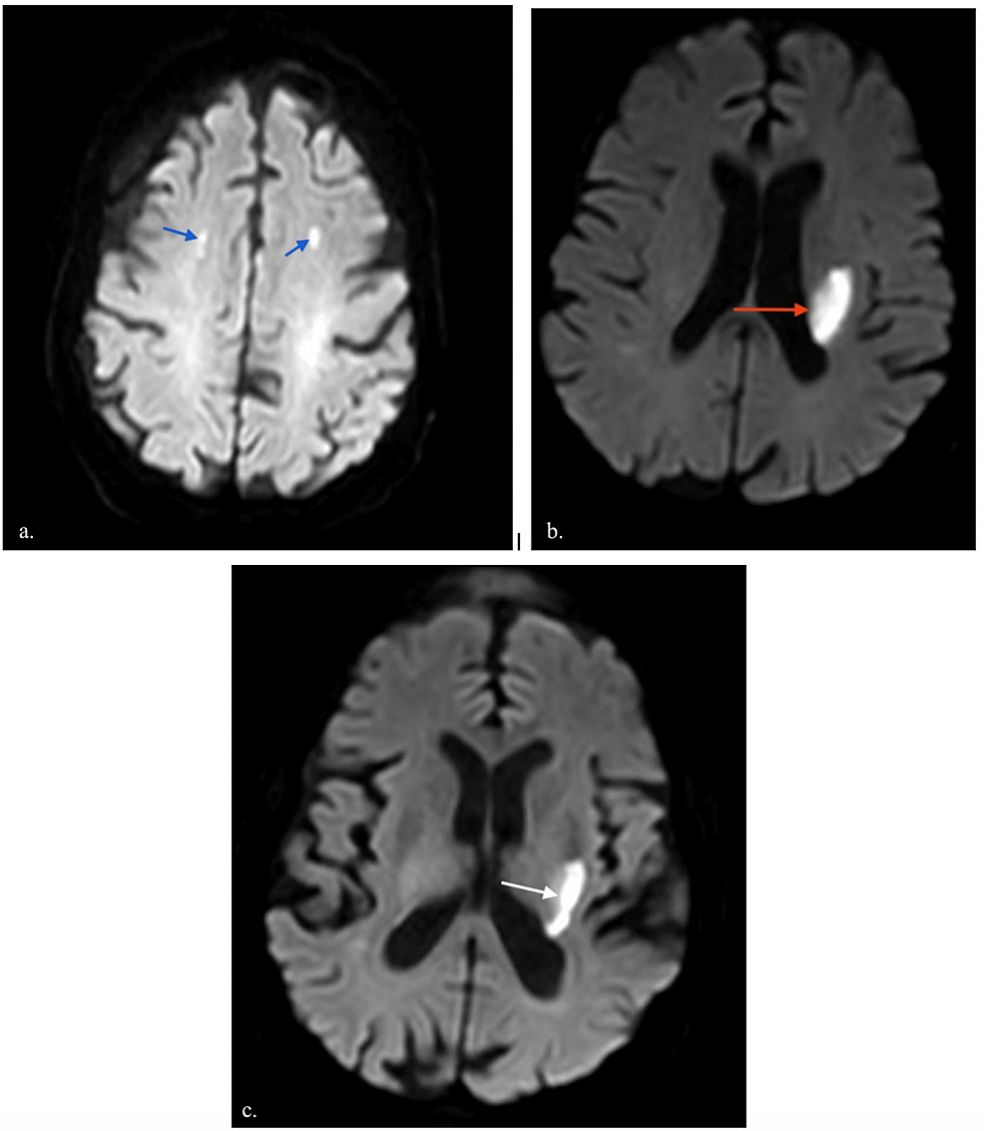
MRI of the brain (a) Diffusion-weighted sequence imaging demonstrated punctate foci of restricted diffusion deep in the white matter of the cerebral hemispheres (blue arrows). (b) There were also restricted diffusion patterns seen in the posterior half of the left internal capsule (red arrow) and the (c) external capsule (white arrow). These widespread patterns indicated embolic events spanning multiple cerebral vascular territories suggesting the showering of emboli consistent with ischemic stroke from a central embolic source, in this case from cardiac valve vegetations MRI: magnetic resonance imaging
